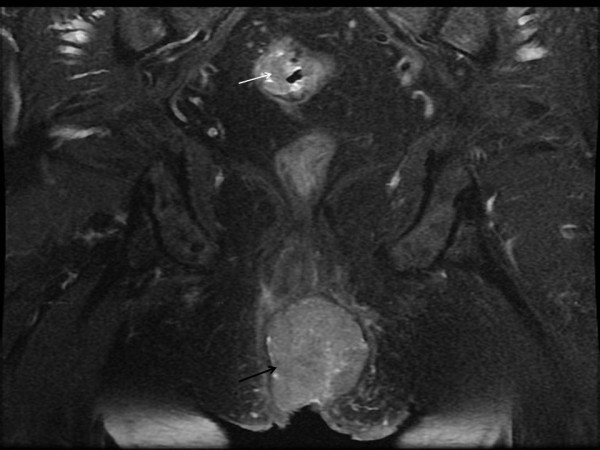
MRI of the pelvis showing the perineal mass (black arrow) and rectosigmoid tumor (white arrow).
